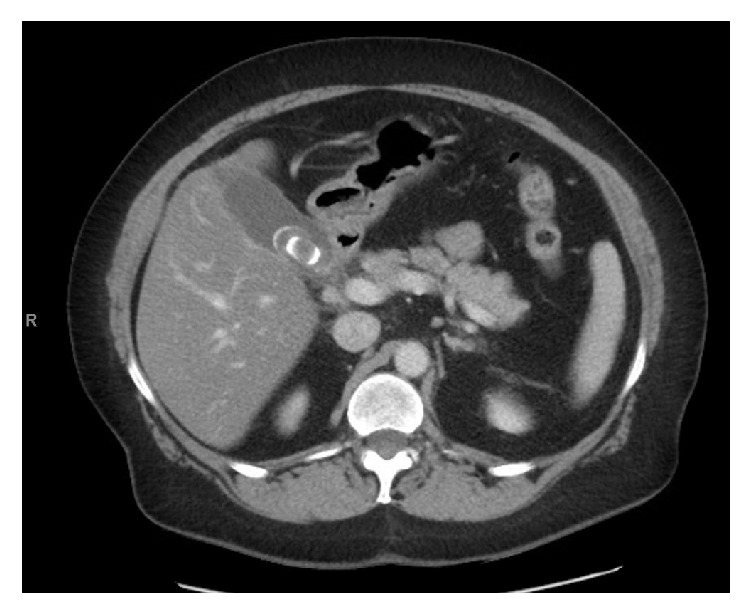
Preoperative CT abdomen with cholelithiasis and duodenal wall thickening.
